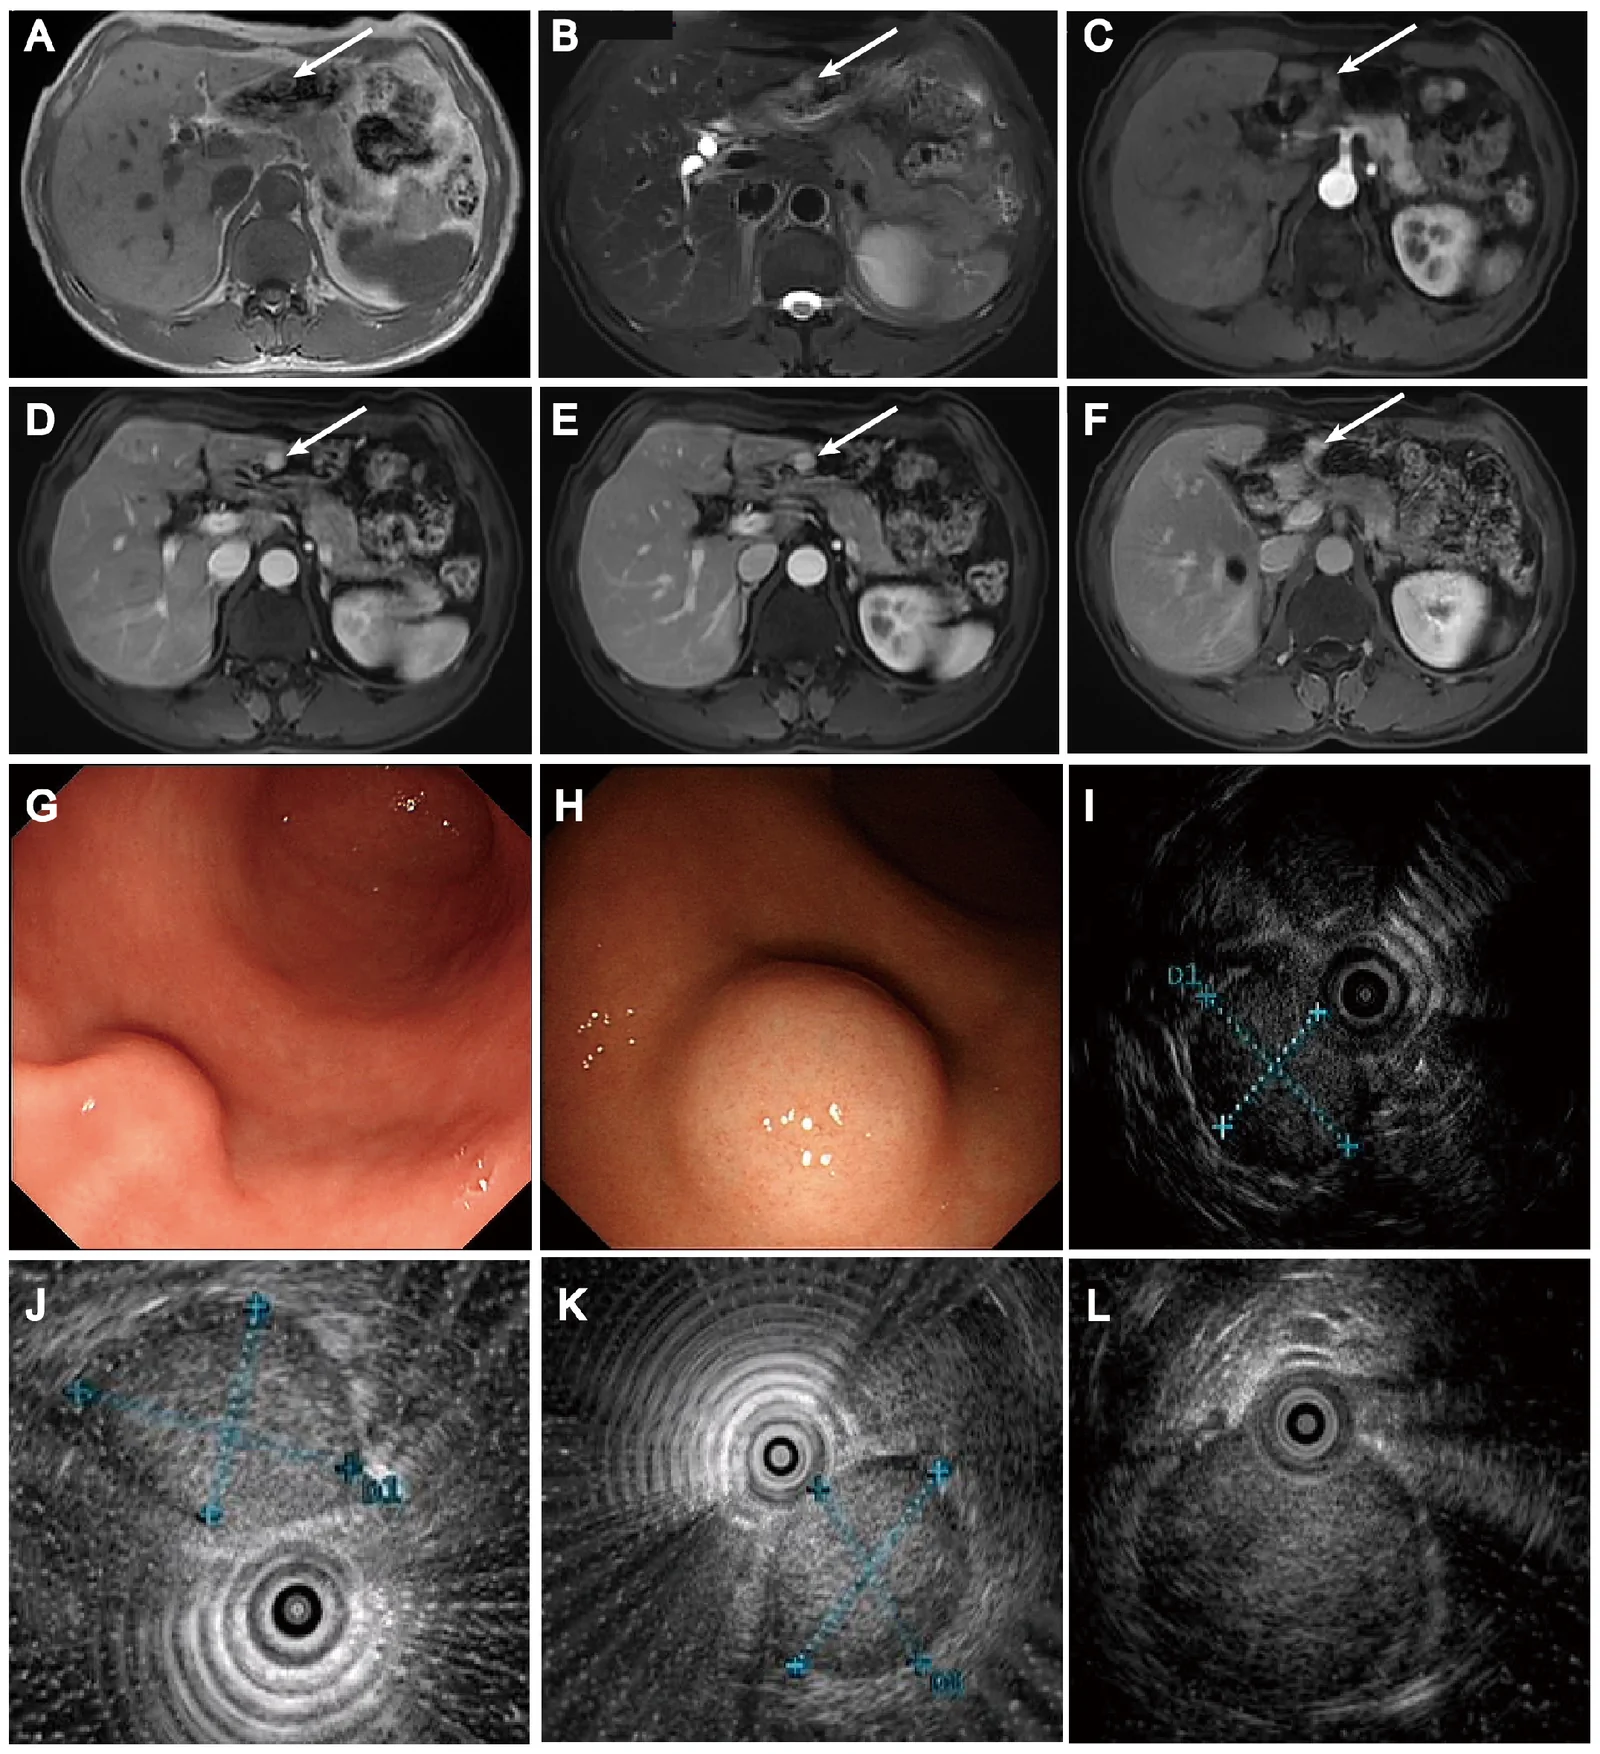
MRI and EUS findings of the lesion. (A) Axial T1WI demonstrates a slightly hypointense lesion in the anterior wall of the gastric antrum. (B) Axial T2WI shows a slightly hyperintense lesion. (C, D) Dynamic contrast-enhanced MRI demonstrates mild enhancement of the lesion in the early arterial phase and progressively increased enhancement in the late arterial phase. (E, F) The lesion shows persistent enhancement in the portal venous and delayed phases. The lesion is indicated by the white arrow. (G, H) Conventional endoscopic examinations performed in 2019 and 2025 demonstrate a persistent subepithelial lesion in the gastric antrum. (I–L) Serial EUS images obtained from 2019 to 2025 show a lesion arising from the muscularis propria. Over this period, the lesion showed an overall increase in size and changed from a homogeneous hypoechoic appearance to a heterogeneous hypoechoic appearance.
